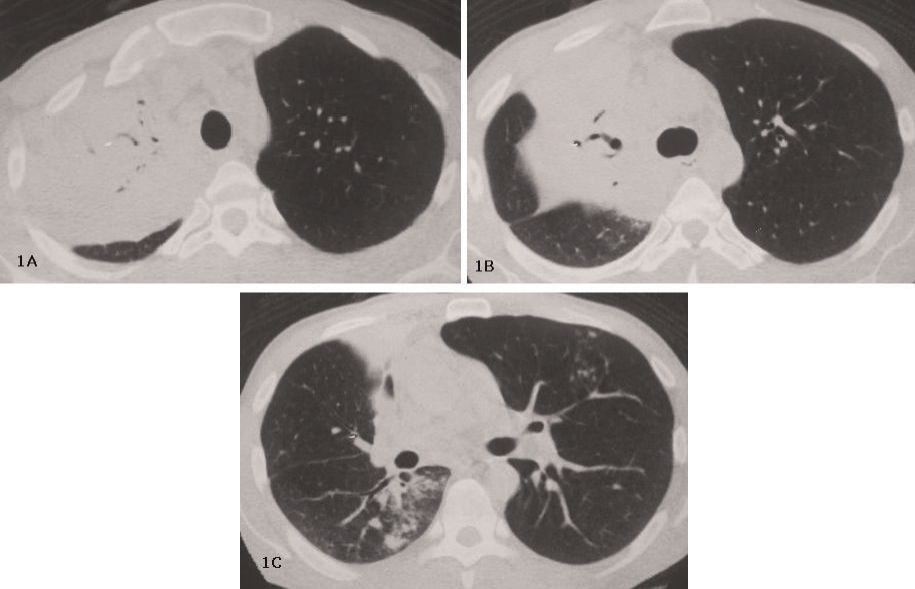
Computed tomography showing extensive area of consolidation with air bronchogram in the right upper lobe. Also note the presence of confluent airspace nodules in the lower part of the consolidation and in the lingular segment (C).
